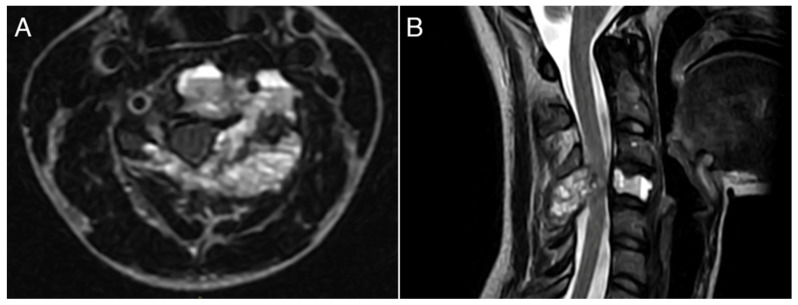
A 26-years-old woman presented with neck pain and no neurological problems. MRI pattern was pathognomonic for ABC. (A): axial view; (B): sagittal view.
